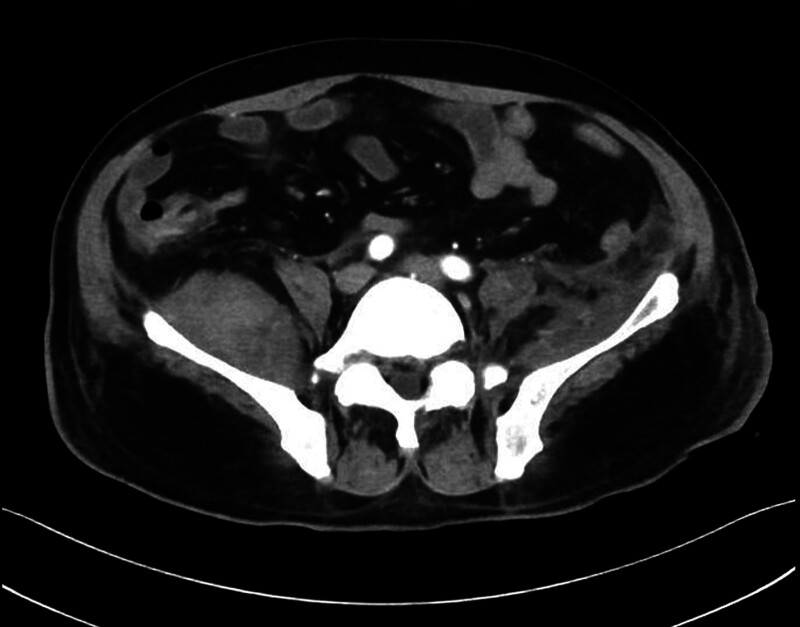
CT scan showed iliopsoas abscess. CT = computed tomography.
